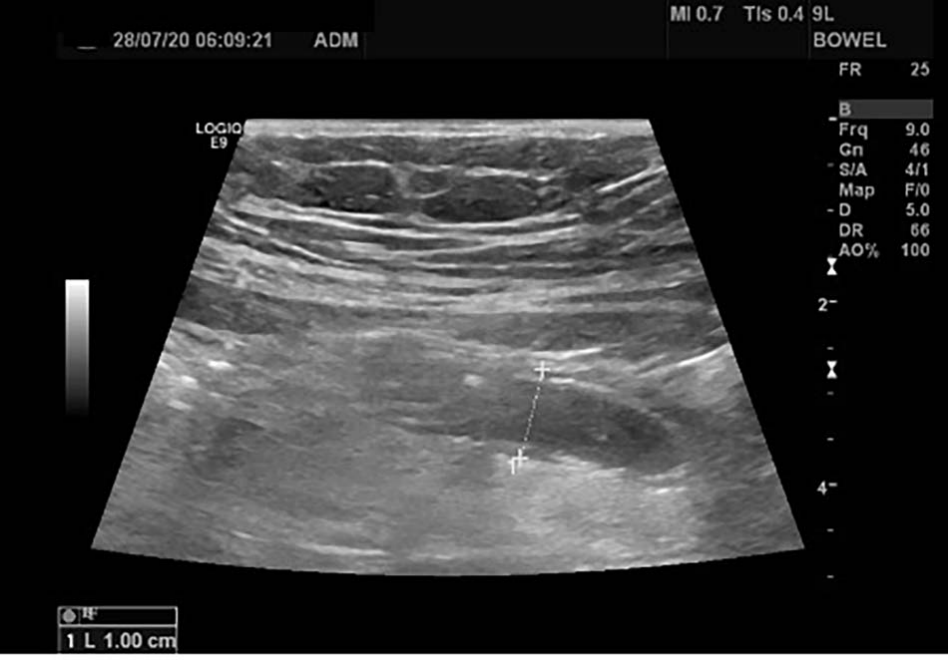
Abdominal ultrasound: The appendix is enlarged (diameter 1 cm) and non-compressible. Inflammation of the adjacent fat is also observed.
